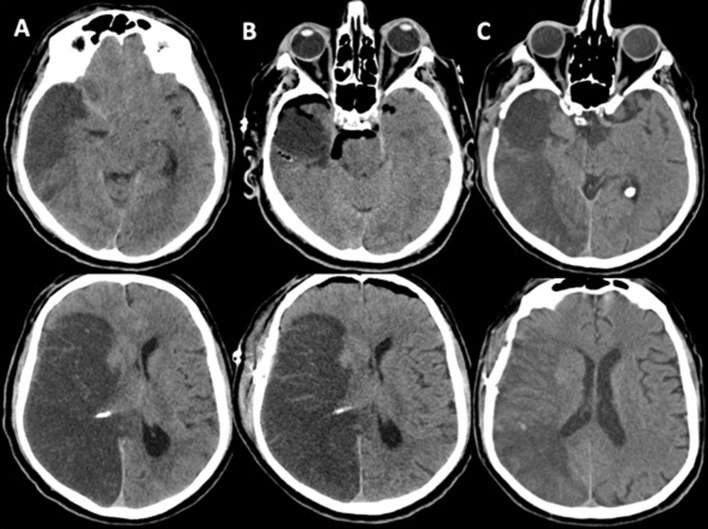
As an example we report the case of a 66-year-old man with extensive MCA stroke. (A) Preoperative CT-scan after clinical deterioration showing trend toward transtentorial herniation and severe midline shift; (B) immediate postoperative CT-scan showing removal of antero-basal portion of right temporal lobe, cisternotomy with extensive CSF drain and relaxation of both hemispheres with bilateral frontal air level; normally after this procedure the midline shift remain evident despite the patient shows clinical improvement: this aspect should be considered in postoperative course; (C) CT-scan on day 15th showing progressive resolution of edema and mass effect; no signs of hydrocephalus are evident. The patient recovered to mRS 3.
